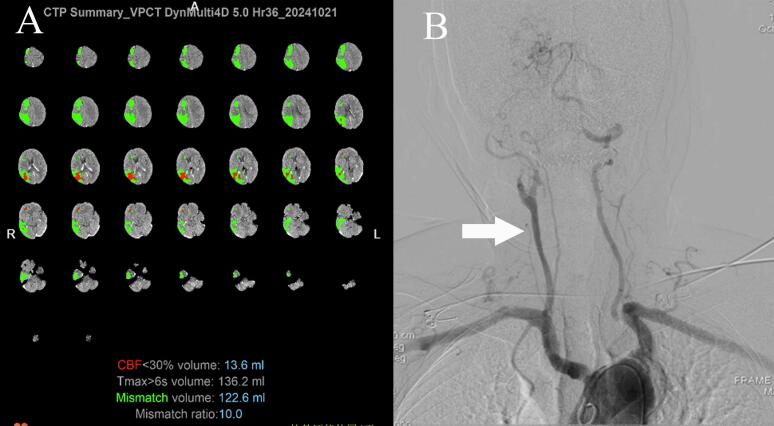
(A) Computed tomography perfusion (CTP) scan identified a significant ischemic penumbra (136.2 ml Tmax >6 s) in the right middle cerebral artery (MCA) territory, with a core infarction volume measuring 13.6 ml; (B) Angiographic finding showed complete occlusion of the right middle cerebral artery (MCA) M1 segment (white arrow).
